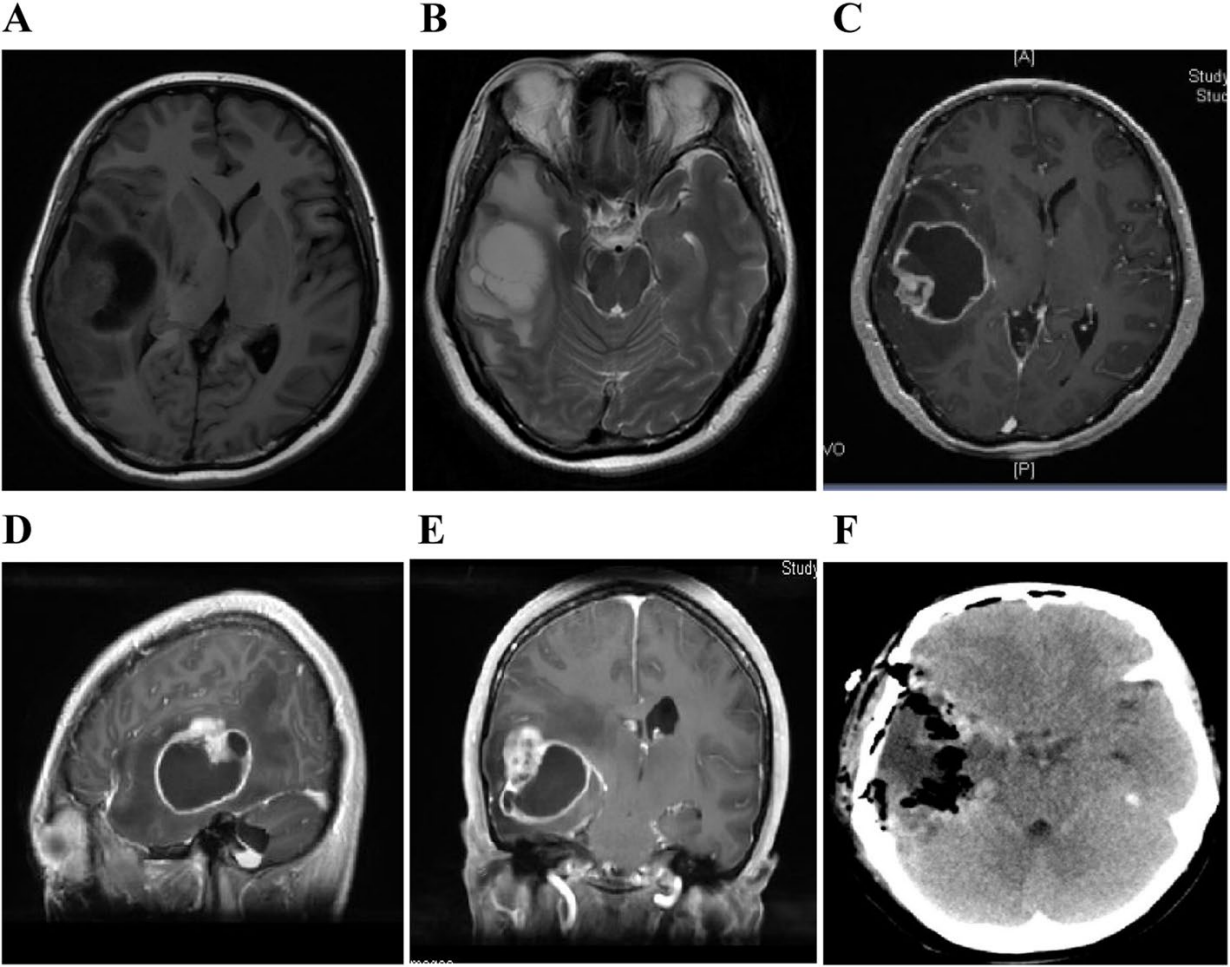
The first preoperative magnetic resonance images of the patient showed a solid cystic space in the right temporal lobe. A T1 phase showed a long and equal T1 signal occupying lesions in the right temporal brain, and the midline was squeezed and shifted to the left. B T2 phase showed a long T2 signal shadow that was larger than the actual occupying lesion. The edema was significant around the lesion. C Axial enhancement. D Sagittal enhancement. E Coronal enhancement all showed solid cystic tumor in the right temporal brain, nodular enhancement of the solid part, and ring enhancement of cystic lesions. F The postoperative CT showed that the tumor was completely removed, without bleeding in the local surgical field, and midline shift was restored
